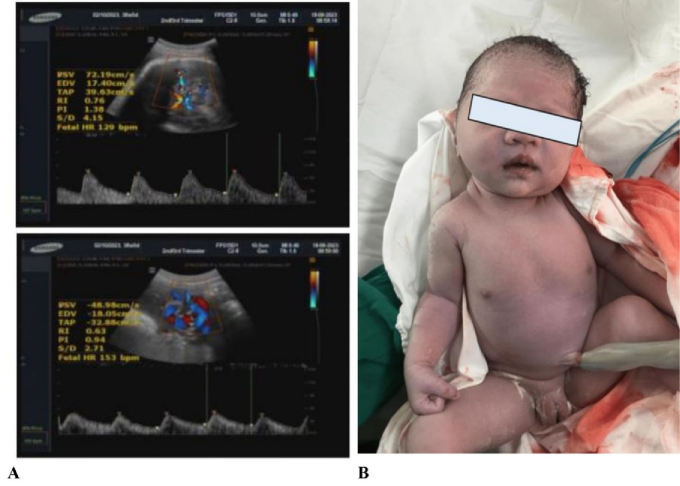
Doppler ultrasound was normally detected before cesarean section (A). A healthy newborn baby was delivered from a TS pregnant woman receiving oocyte donation (B).
